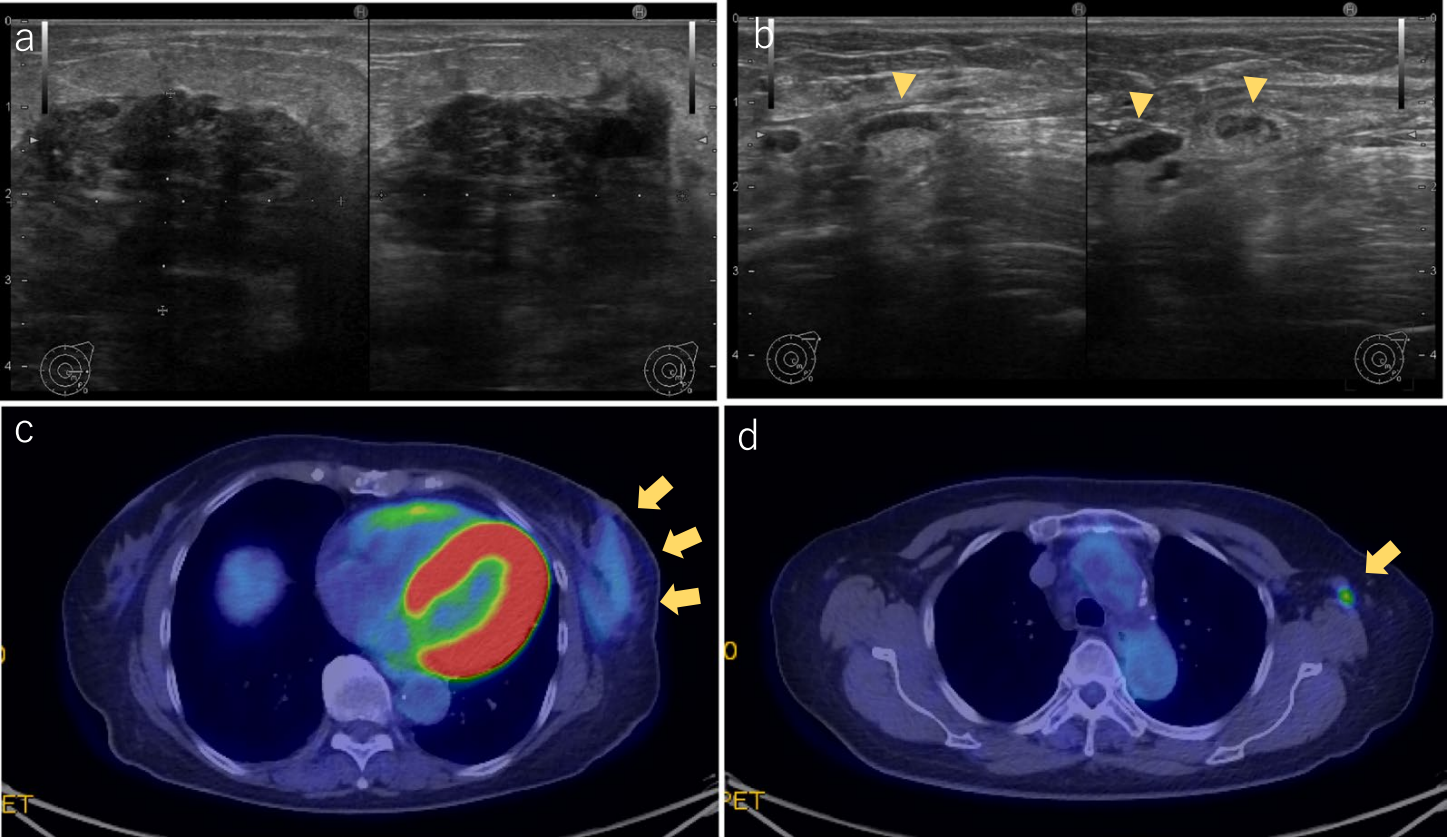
Clinical imaging findings at initial examination. a Ultrasound of the breast: a 3.8 × 2.5 × 3.5 cm large non-mass lesion from below the nipple to the 3 o'clock position. b Lymph nodes with cortical thickening in the left axilla (arrowhead). c, d PET-CT: SUVmax 2.1 in the left mammary gland and SUVmax 3.5 in the left axillary lymph node (arrow). PET-CT, positron emission tomography-computed tomography
